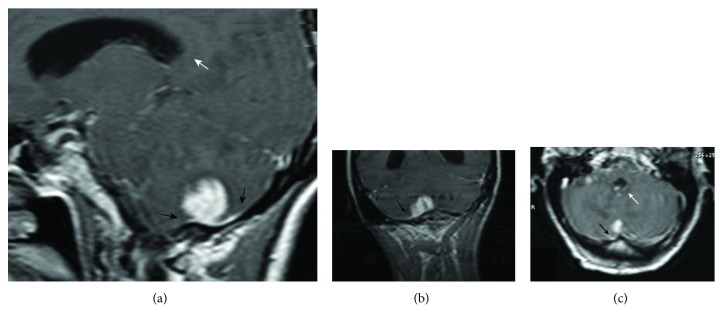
Preoperative T1 postgadolinium injection MRI (a) sagittal, (b) coronal and (c) axial, showing a meningioma-like lesion, localized at the base of the posterior cranial fossa and reaching the cortex of the right cerebellar hemisphere. Note the dural tail (black arrow) and the dilatation of the ventricular system (white arrow).
